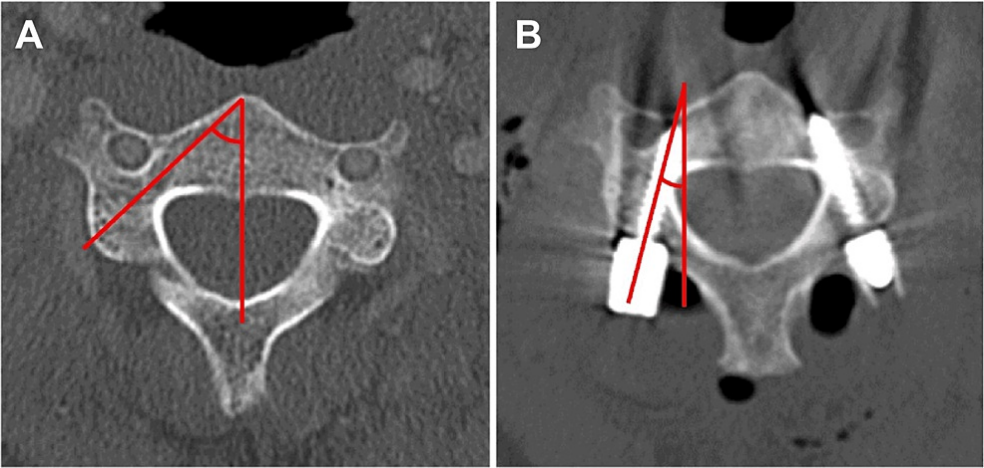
Preoperative and postoperative computed tomography (CT). The anatomical medial angle of the pedicle (A) was measured using preoperative CT. The actual angle of the screw (B) was measured using postoperative CT.
